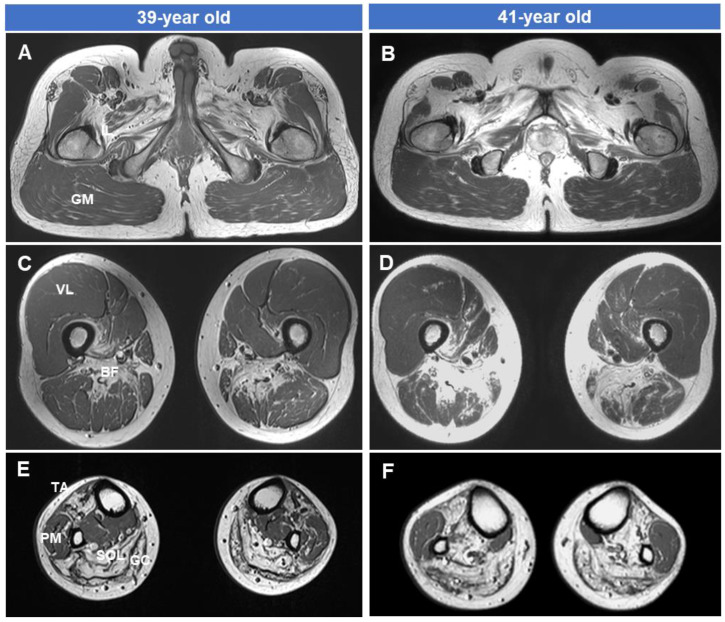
T1-weighted lower extremity MRIs in the male patient of the Myo-41 family. Axial images of the hip (A,B), thigh (C,D), and lower leg (E,F) were taken at 39 (A,C,E) and 41 (B,D,F) years of age. Note the severe fatty involvement of the biceps femoris short head (BF) muscles at the thigh level and the tibialis anterior (TA), soleus (SOL), and gastrocnemius (GC) muscles at the lower leg level. However, the vastus lateralis (VL), gluteus maximus (GM), and peronei muscles (PM) are selectively preserved. This MRI study, conducted over two years, showed rapid progression of muscle degeneration and fat replacement.
